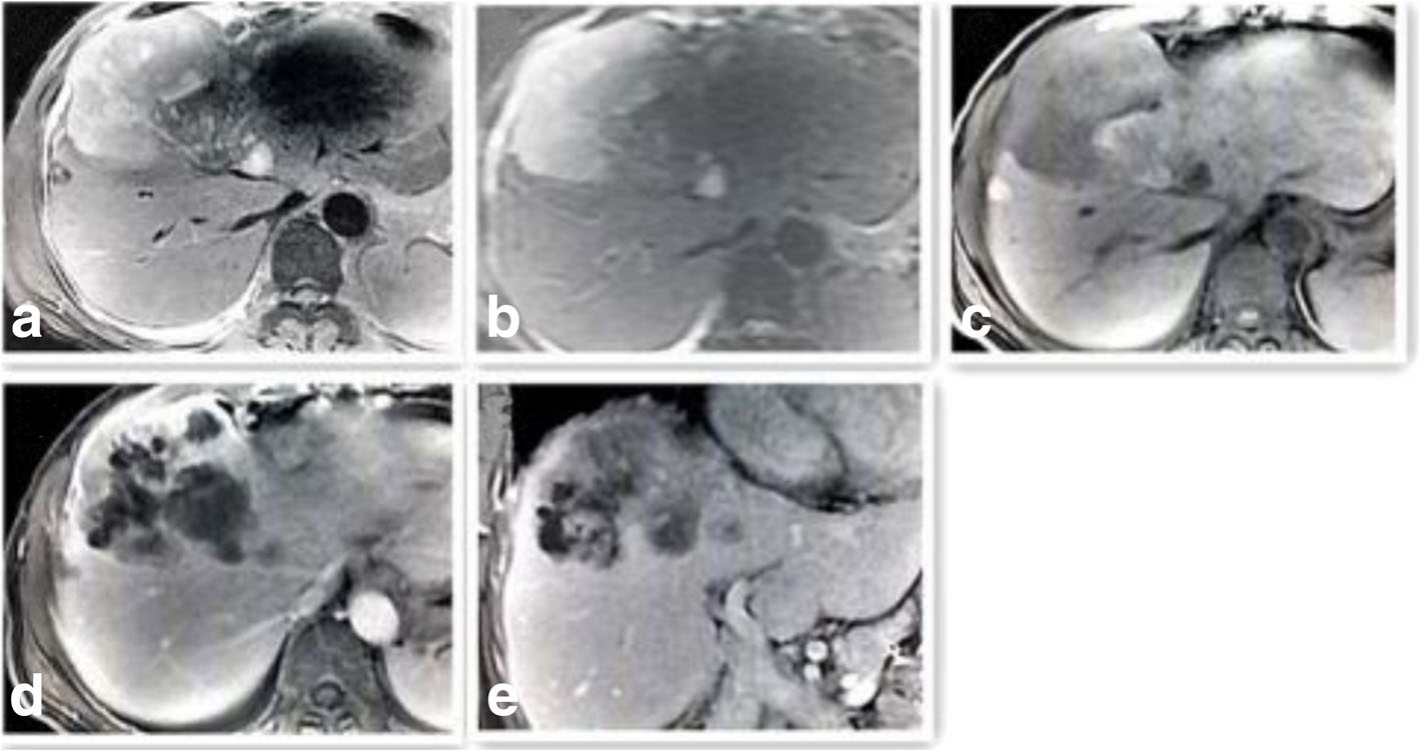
MR images (a-e). T2-weighted axial (a) and diffusion (b = 800) (b) images showing well-circumscribed areas, high signal intensity, and irregular large low signal intensity masses in the right liver lobe. T1-weighted image (c) showing well-circumscribed, heterogeneous, and hypointense masses. Irregular enhancement and no enhancement lumps were observed in the early arterial phase (d) and 5 min delayed hepatobiliary phase (e)
